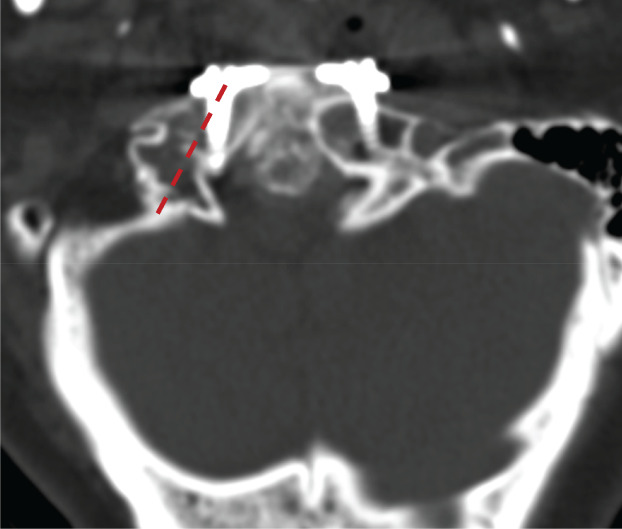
Misplacement of screws in the atlas. Postoperative CT scan shows that part of the screw (the right side) was inserted into the spinal canal. The red line shows the ideal screw path.
